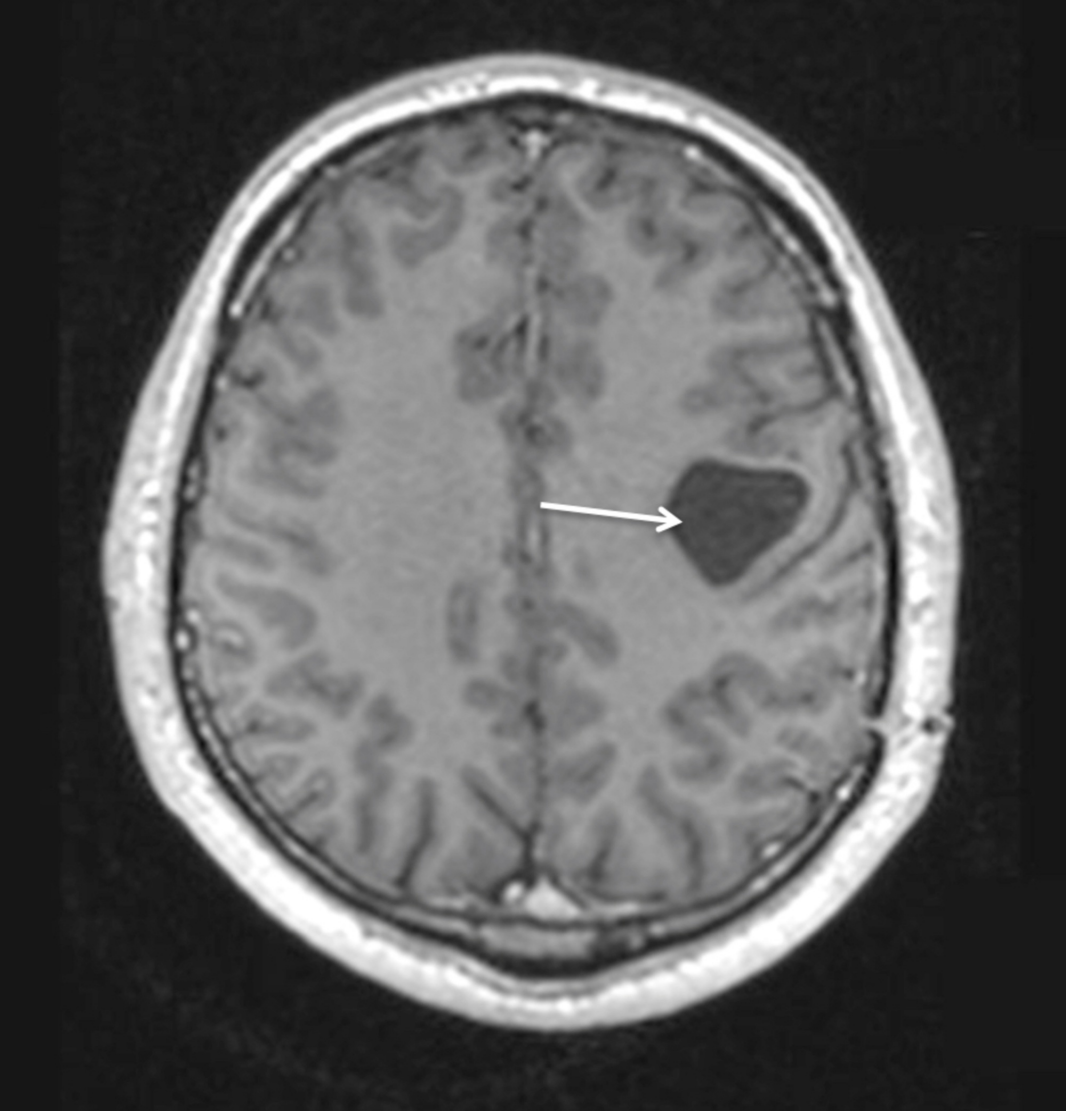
Postoperative MRI with contrast enhancement at eight months follow-up demonstrating the residual cyst without contrast accumulation (arrow), decrease in size, and no mass effect on the T1WI axial view. MRI: Magnetic resonance imaging; T1WI: T1-weighted images.
